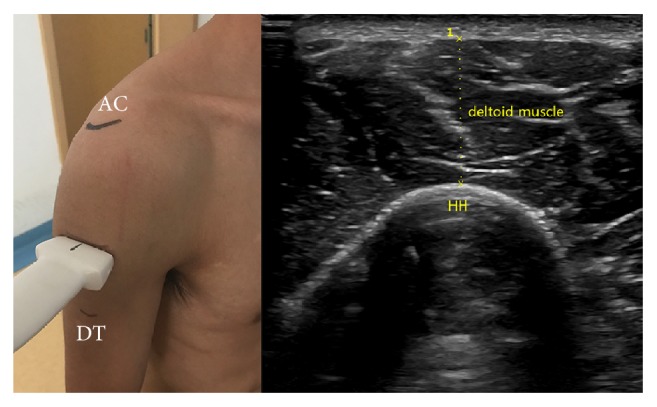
Anatomical structures: DT (deltoid tuberosity); the position and orientation of transducer; and the ultrasound image: DMT (deltoid muscle thickness).
